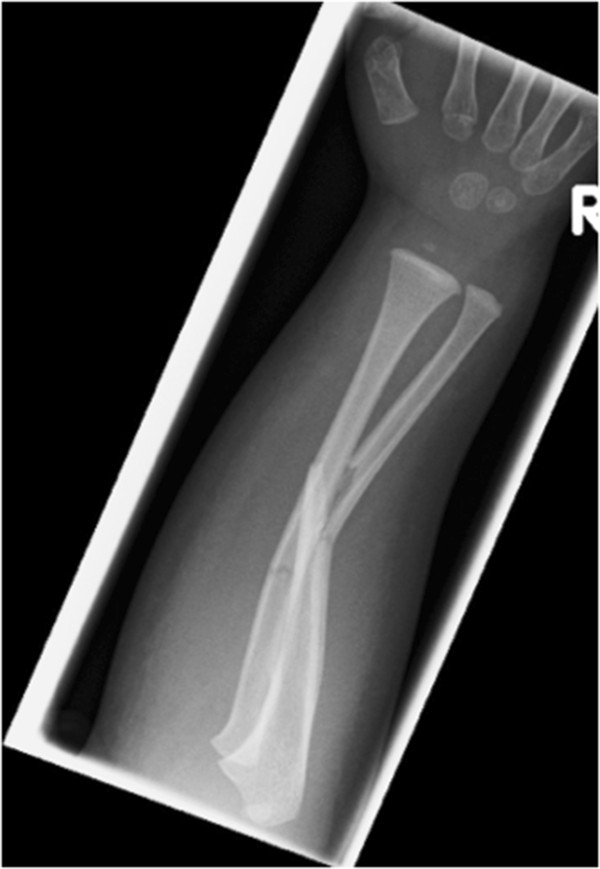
Pre-operative radiographs demonstrating displaced both bone forearm fractures.
